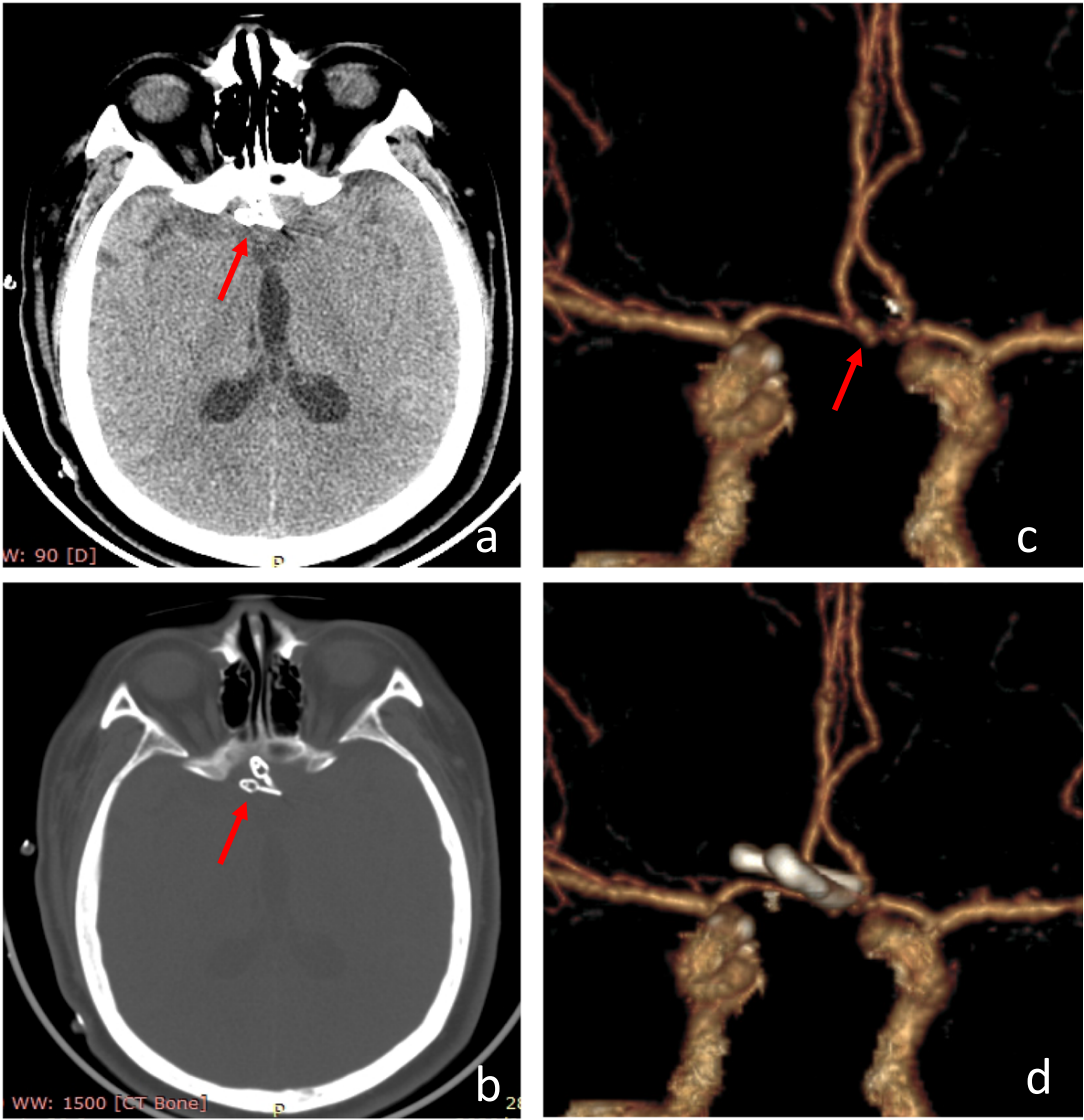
Preoperative and postoperative imaging examination and intraoperative findings of ruptured anterior communicating aneurysms with reconstruction clipping via supraorbital lateral keyhole approach. a, b On postoperative CT, there were 2 aneurysm clips at the arrow; c The shape of anterior communicating artery aneurysm at the arrow is irregular; d Postoperative reexamination showed that the aneurysm was completely clipped and other blood vessels were not damaged
